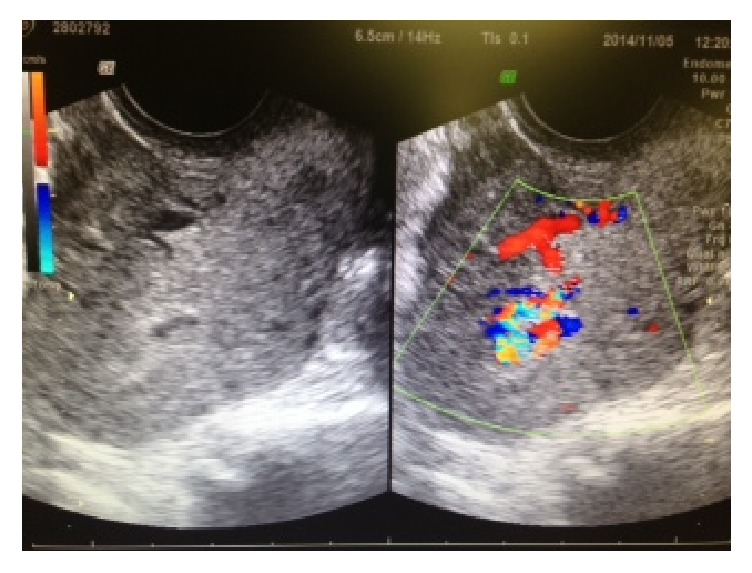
AVM depicted by ultrasonography using grayscale and color Doppler (Patient 3, mass measuring 20 × 15 mm in size).
